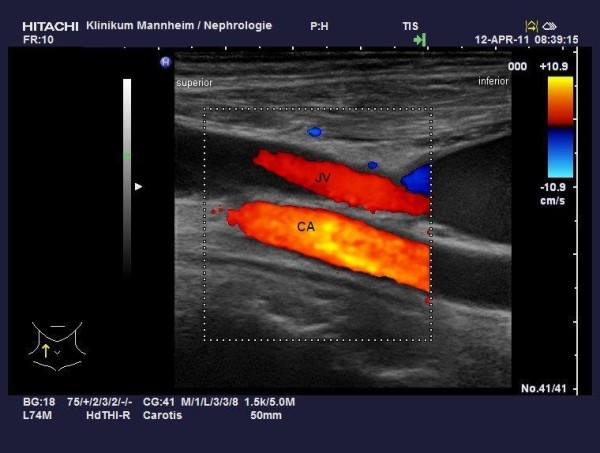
Color duplex sonography of the right jugular vein and carotid artery showing a cranial flow direction in both.
